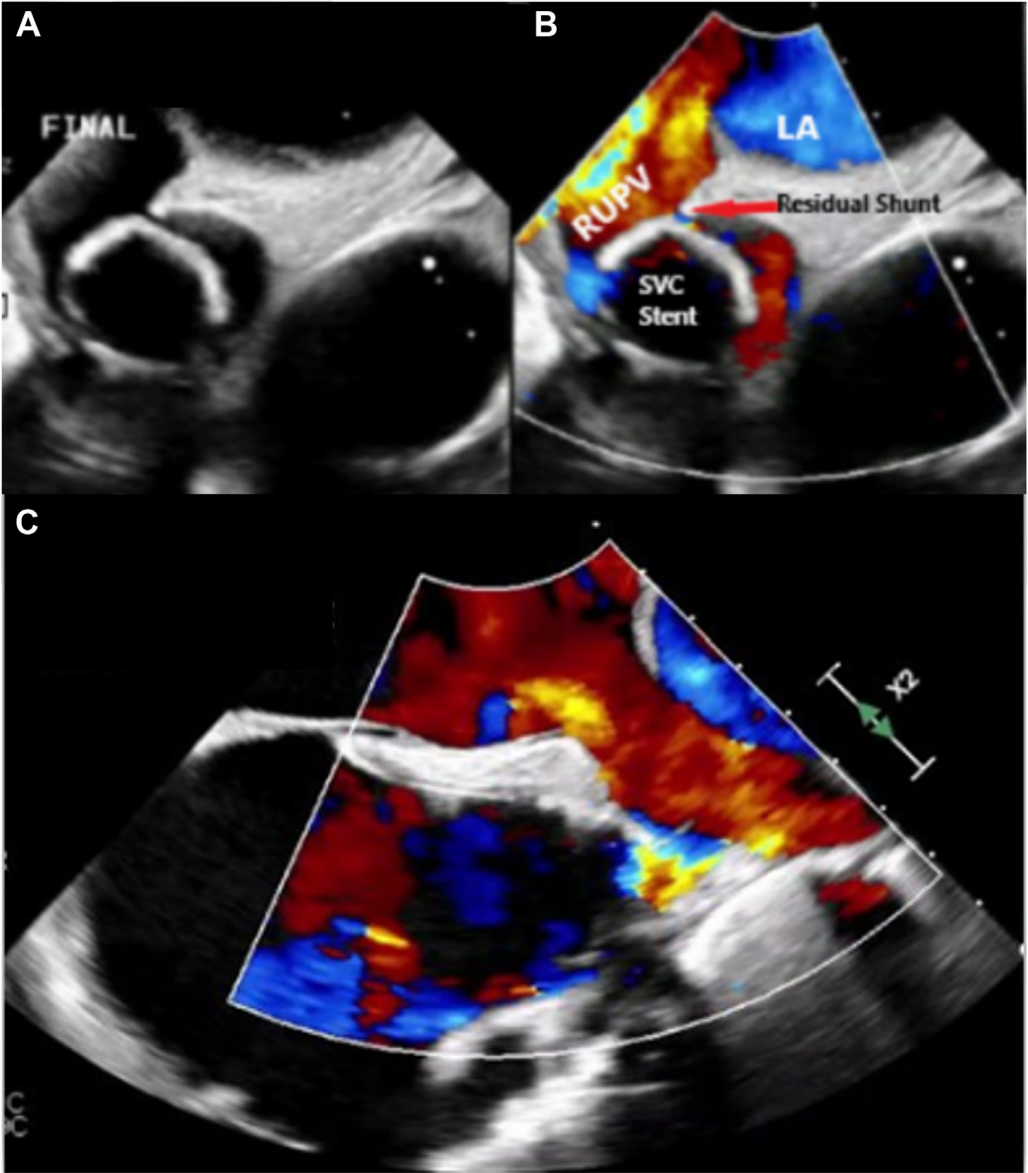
Intraprocedural Transesophageal Echocardiography of Sinus Venosus Atrial Septal Defect With Partial Anomalous Pulmonary Venous Return Closure Successful redirection of the anomalous right-sided pulmonary veins to the left atrium (LA). Stable HTPV22 frame from the SVC into the superior vena cava (SVC)–right atrial junction. RUPV = right upper pulmonary vein.
